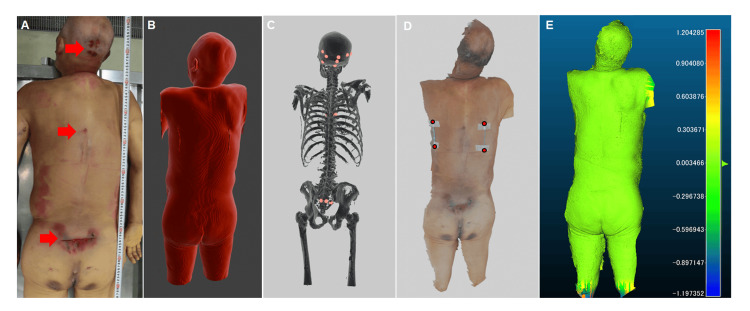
Photographs and 3D model of the body. (A) Photograph taken during the autopsy. Abrasion and subcutaneous hemorrhage can be observed in the head, midspine, and sacral regions. (B) Skin surface model reconstructed from postmortem CT (PMCT) data. (C) Bone model reconstructed from PMCT data. The red spheres indicate fracture locations. (D) Surface 3D model of the skin generated using photogrammetry. The catheter tubes were placed below the scapulae. Alignment between the PMCT model and the surface 3D model was performed using red markers. (E) Alignment analysis using CloudCompare. Green indicates a high degree of similarity between the two models, whereas red and blue indicate areas of greater discrepancy.
